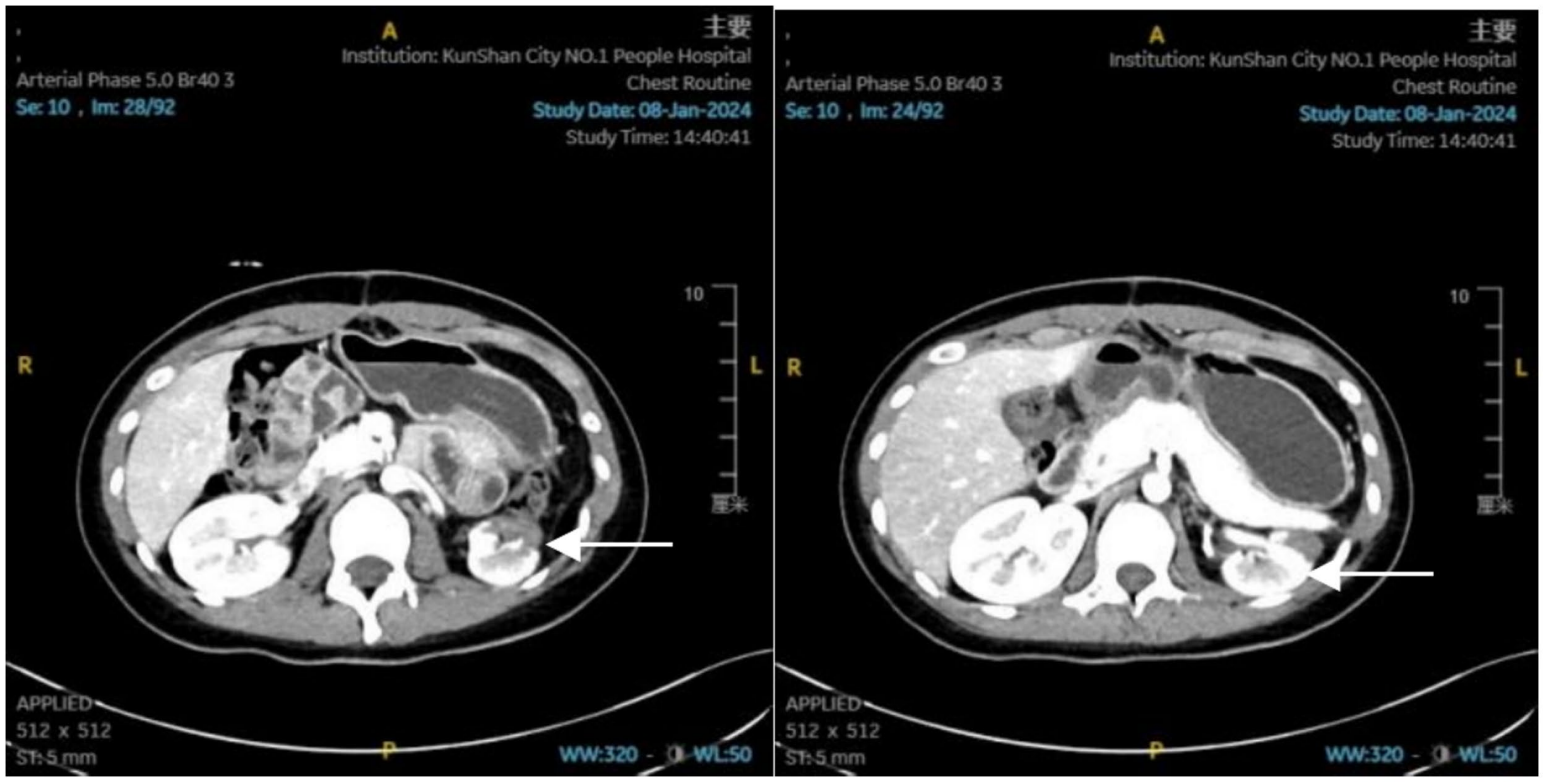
Abdominal enhanced CT report: status post-splenectomy; contusion of the left lobe of the liver, which has shown significant improvement. Contusion of the left adrenal gland, also showing significant improvement. Slight atrophy of the left kidney.
